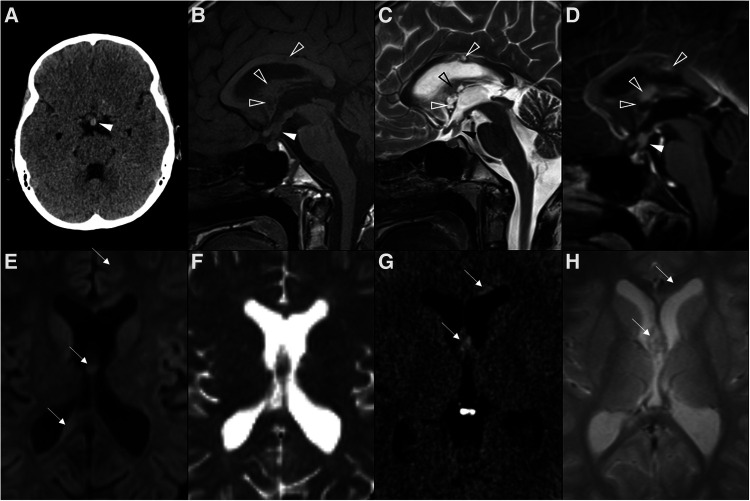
Multifocal germinoma in a 12-year-old boy with panhypopituitarism. Thickening of the pituitary stalk and tuber cinereum was observed in axial head CT (A) and sagittal T1WI (B) and T2WI (C), which enhanced homogenously after gadolinium in sagittal T1WI (D) (white arrowheads). The expected T1 hyperintensity of the neurohypophysis is absent. Also note additional solid-cystic lesions in the body of the corpus callosum, lamina terminalis, and ceiling of the third ventricle with associated contrast enhancement (empty white arrowheads), indicative of multifocal distribution. Despite no diffusion restriction was seen on DWI (E) and ADC map (F), all lesions were spontaneously hyperdense on CT (G) with no hypodense foci on T2 gradient-echo images (H), supporting some degree of hypercellularity (white arrows).
